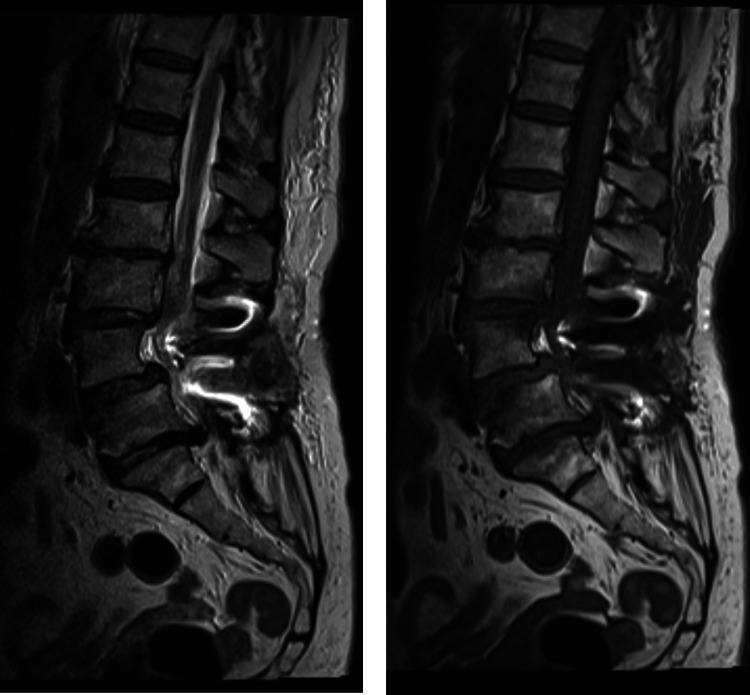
Post-operative lumbar images The MRI was unable to document liquor leak points.
